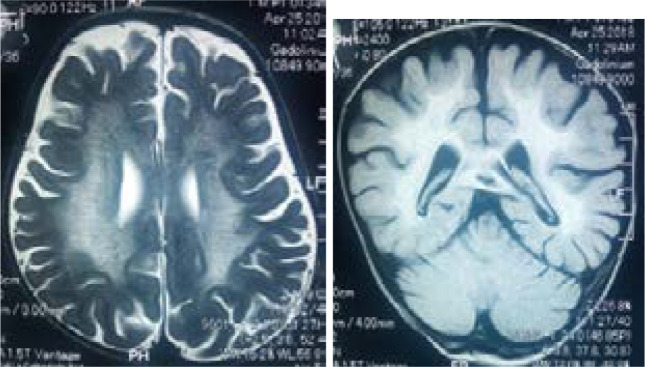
Brain MRI without contrast injection in axial section T1, T2 and FLAIR sequence: T2 and FLAIR hyperintensity of the deep white matter bilaterally and symmetrically and T1 hyposignal associated with corpus callosum involvement compatible with adrenoleukodystrophy
